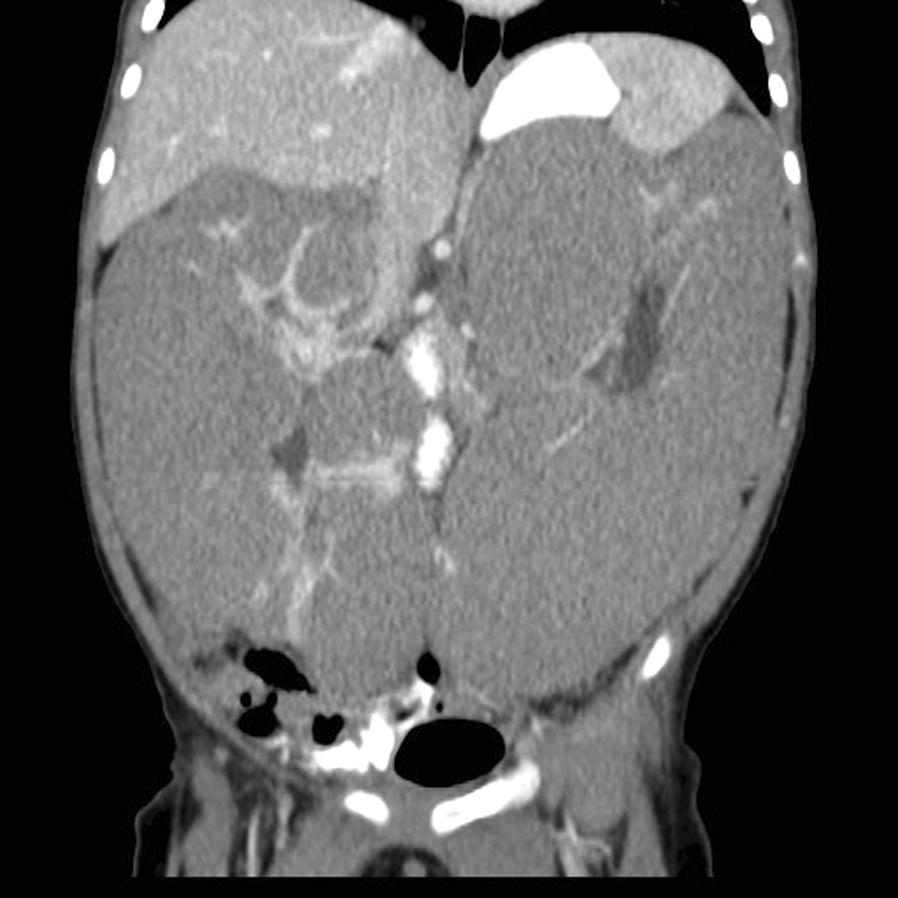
Coronal slice of CT abdomen/pelvis with IV and oral contrast showing bilateral homogenous, diffuse renal enlargement with low-attenuating perinephric nephrogenic rests
